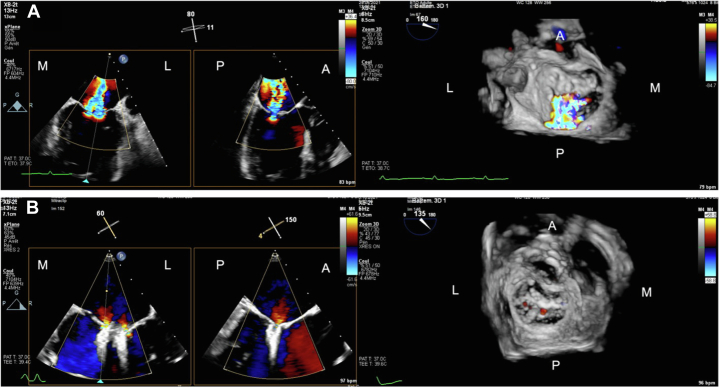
Preprocedural and Postprocedural Transesophageal Echocardiography (A) Preprocedural and (B) postprocedural transesophageal echocardiography showing the optimal result with residual trace regurgitation. A = anterior; L = lateral; M = medial; P = posterior.
